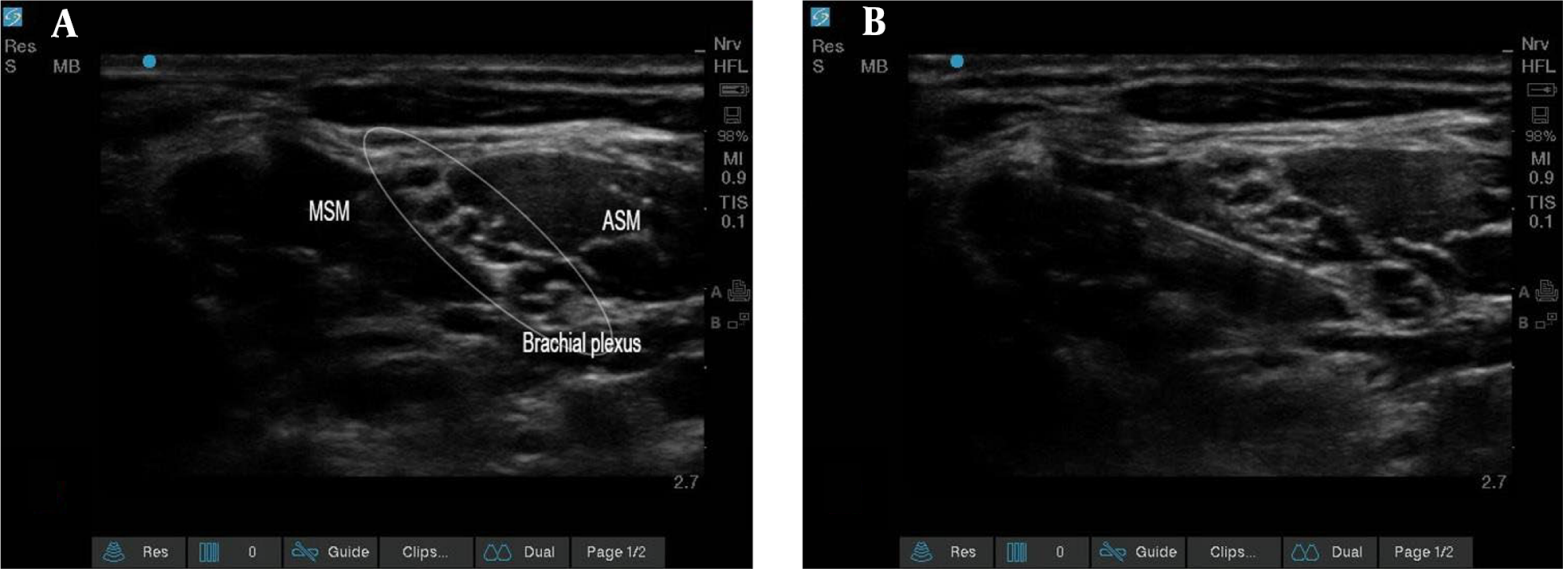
Sonography of the brachial plexus, middle scalene muscle, and anterior scalene muscle. The b and a are before and after the injection, respectively (Abbreviations: MSM, middle scalene muscle; ASM, anterior scalene muscle).
